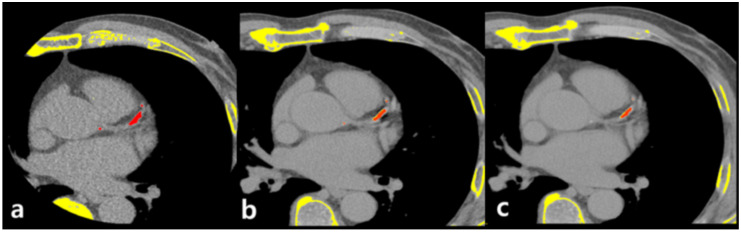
Representative case of coronary calcium scoring on calcium-scoring CT and chest dual energy CT. Pixels with HU > 130 are displayed in yellow and red if selected as calcium by operator. Calcium is well visualized on calcium-scoring CT (a), 1.25 mm slice thickness VUE image of chest DECT (b), and 2.5 mm slice thickness VUE image of chest DECT (c).
